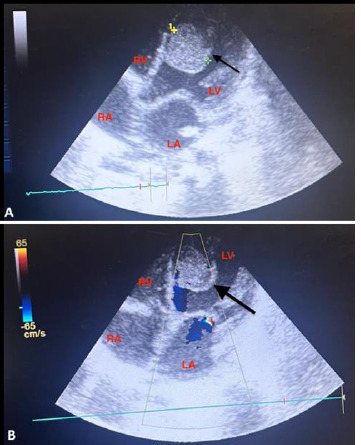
A, B) apical section of cardiac ultrasound showing a giant mass in the left ventricle
